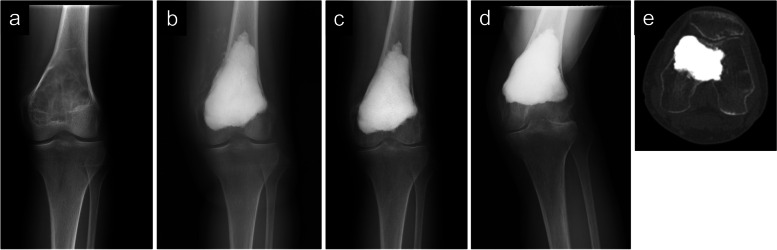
Preoperative radiogram images. Preoperative plain radiograms of the left knee joint showed an osteolytic lesion with scalloping (a). A postoperative plain radiogram showed that the cavity was filled with calcium phosphate cement (b). A plain radiogram after 5 years showed a K-L grade 3 joint space narrowing (c). A plain radiogram after 6 years showed the progressive joint space narrowing; the narrowing by the time of this radiogram was graded as K-L grade 4 (lateral tibiofemoral) and grade 2 (medial tibiofemoral) osteoarthritis (d). A CT imaging showed K-L grade 4 patellofemoral osteoarthritis
